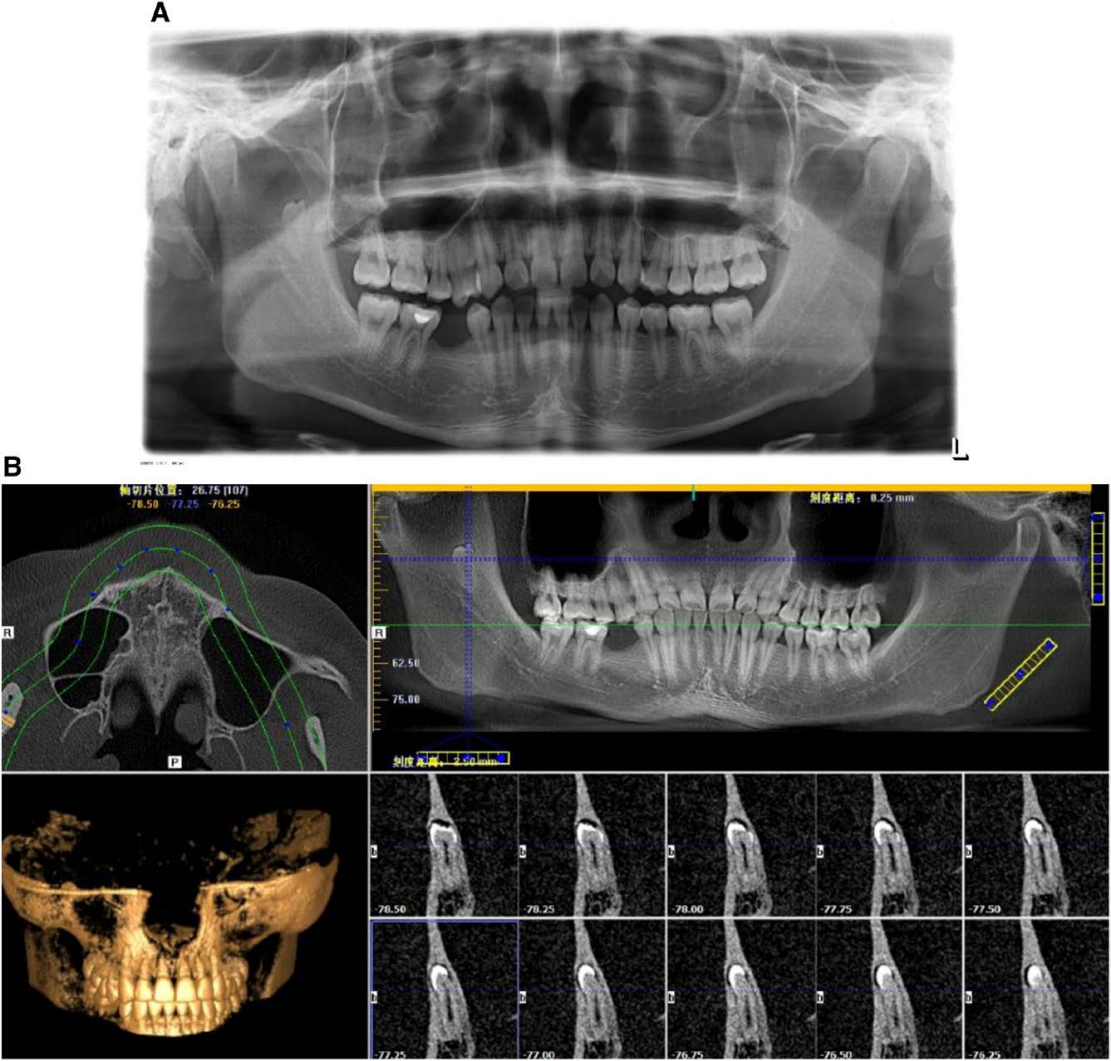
Imaging and dental clinical examination. (A and B) The panoramic radiograph and CBCT of the patient showing an impacted teeth in the Mandibular ramus. CBCT = cone beam computer tomography.
